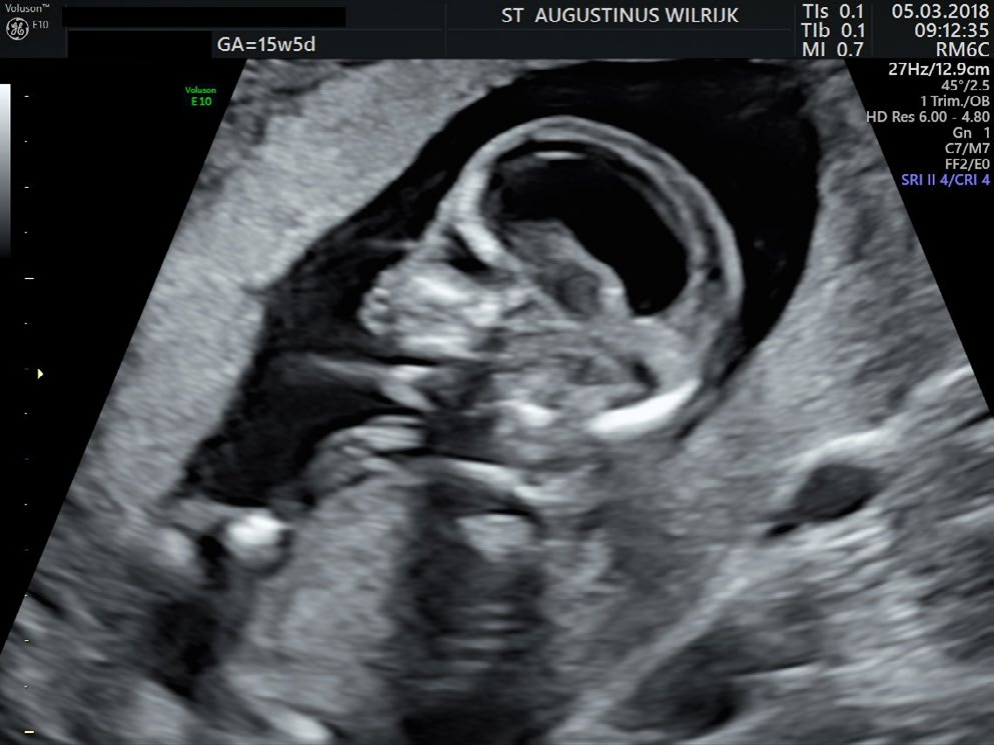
Ultrasound at 15 wks and 5 d shows a more pronounced holoprosencephaly
